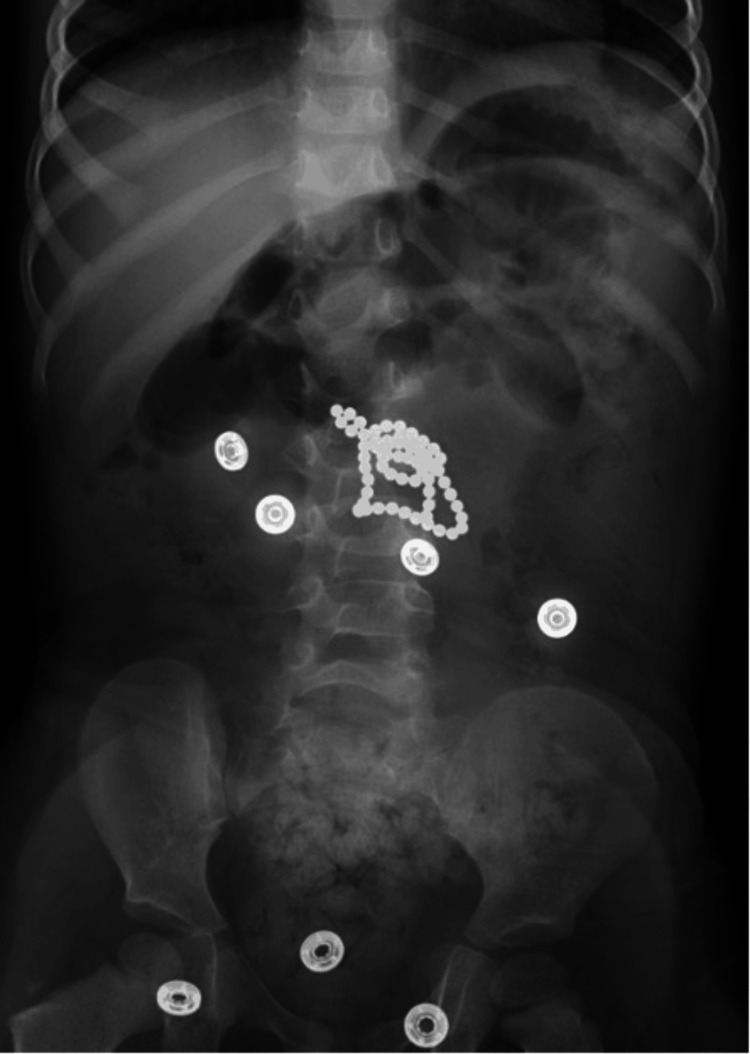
Abdominal X-ray of Foreign Body and Small Bowel Obstruction
